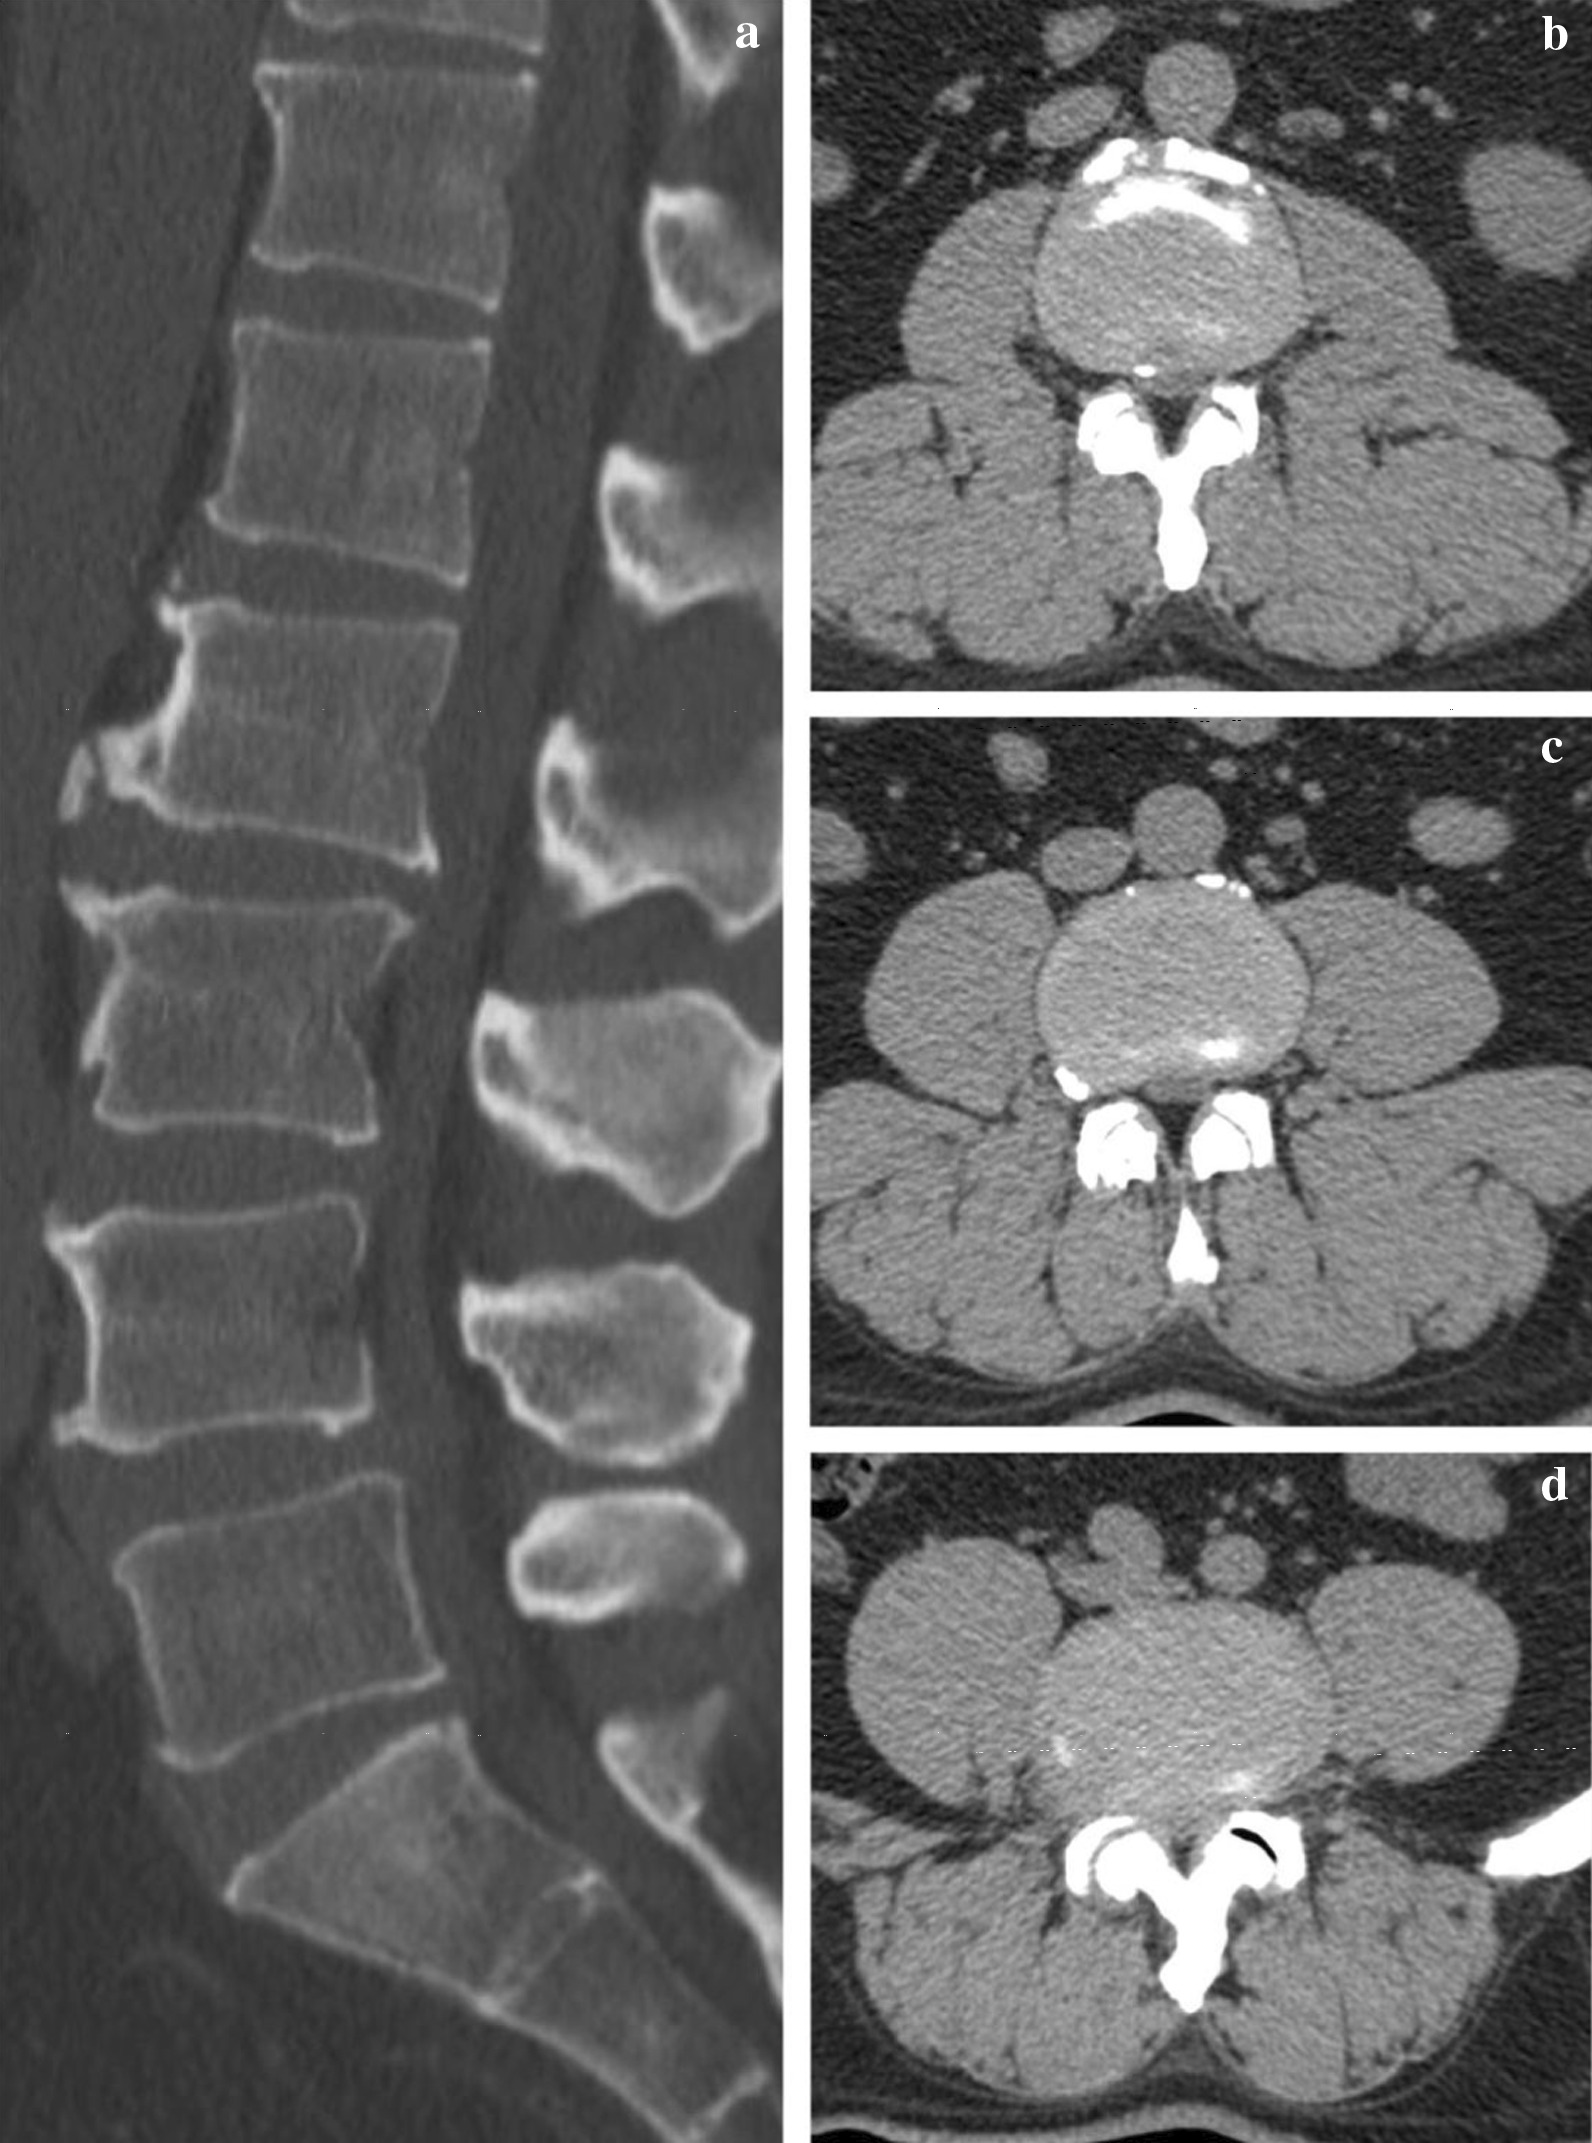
Preoperative CT scan. Preoperative sagittal CT scan indicates intervertebral space narrow, vertebral body bone hyperplasia, “Y” shape homogenious hypodense shadow and oval compression of caudal spinal cord. Axial images shows stenosis frome L2 to L5 and mild hypertrophy of ligamentum flavum and facet joint. a Sagittal image. b Axial image of L2/3. c Axial image of L3/4. d Axial image of L4/5
